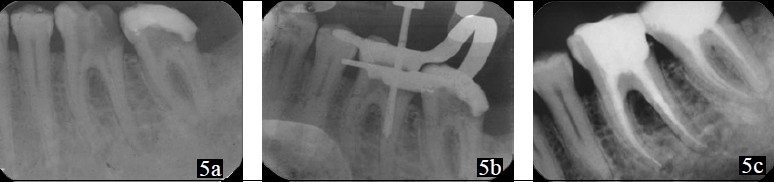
(a) – Radiograph showing the separated file in the distolingual canal; (b) – Extractor with plunger sleeving and gripping the fragment; (c) – Post-obturation 36, 37
